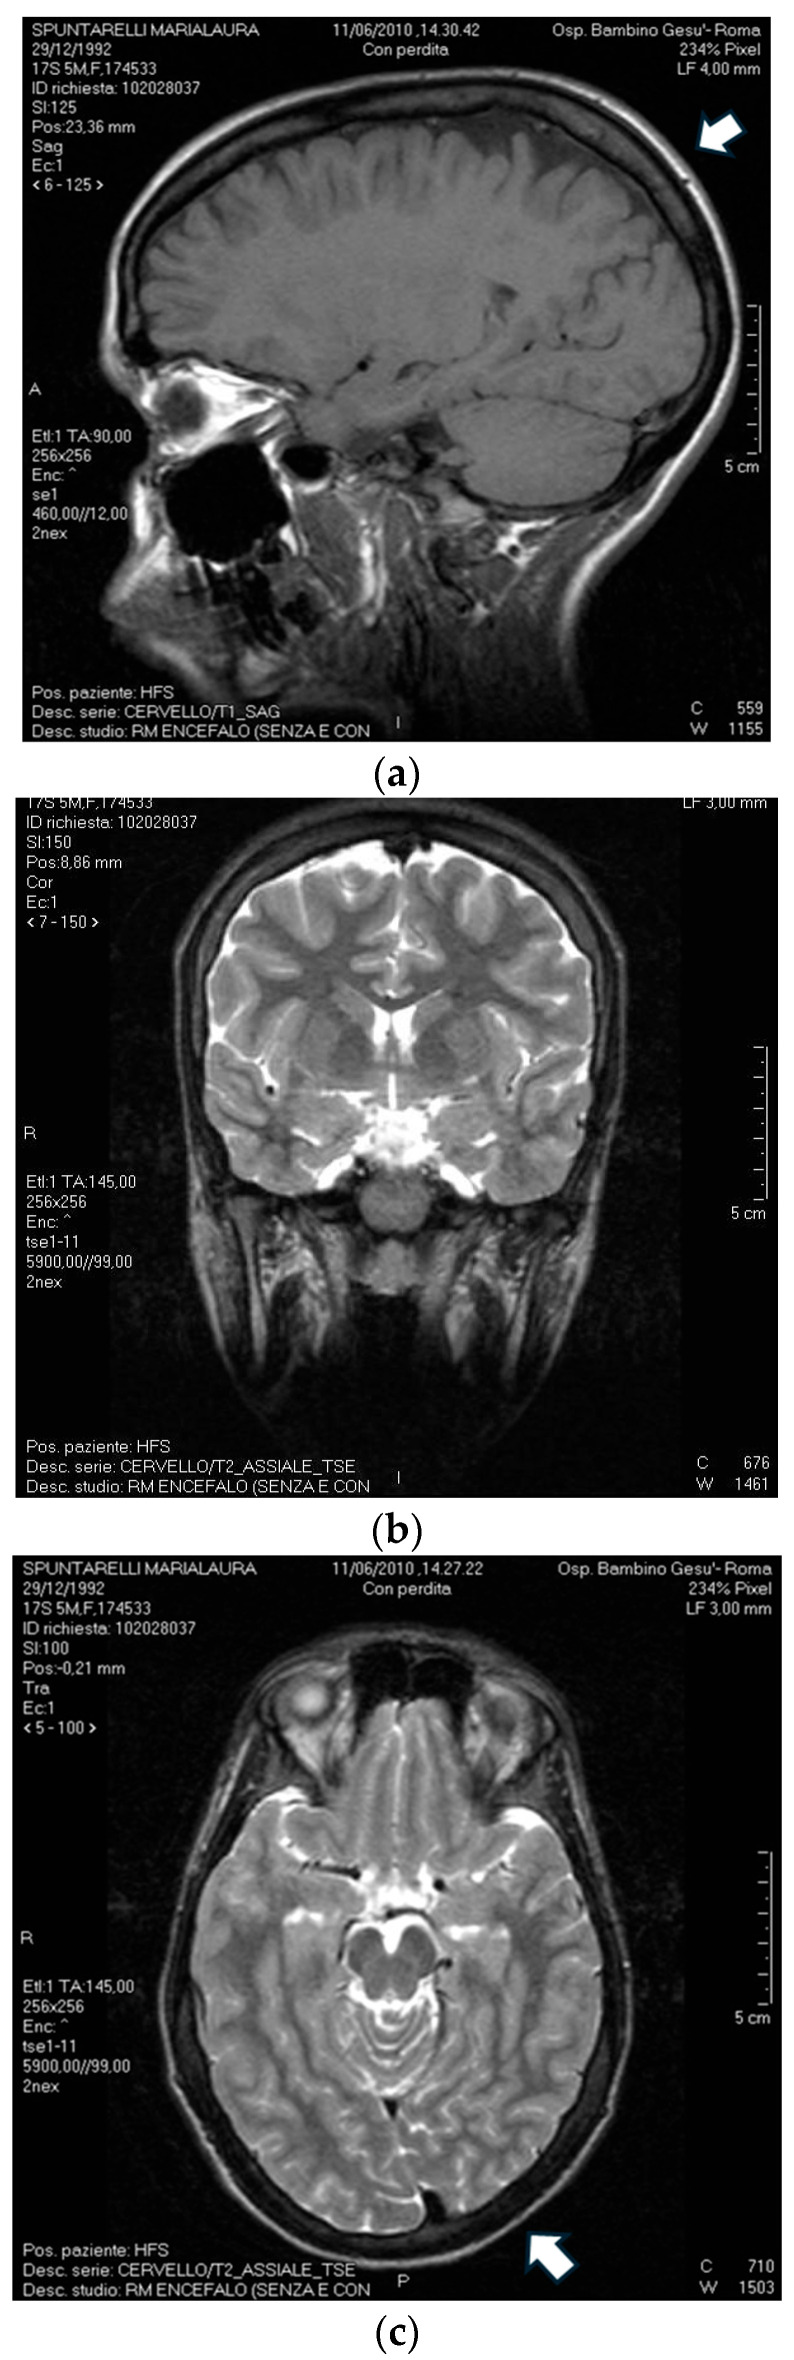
Brain MRI of the proband performed at 18 years of age, with (a) no significant malformation in the sagittal plane, white arrow indicates the small expansion of the periencephalic liquor spaces (b) asymmetry of the occipital lobes in the coronal plane (c) white arrow indicates the asymmetry of the occipital lobes in the axial plane.
